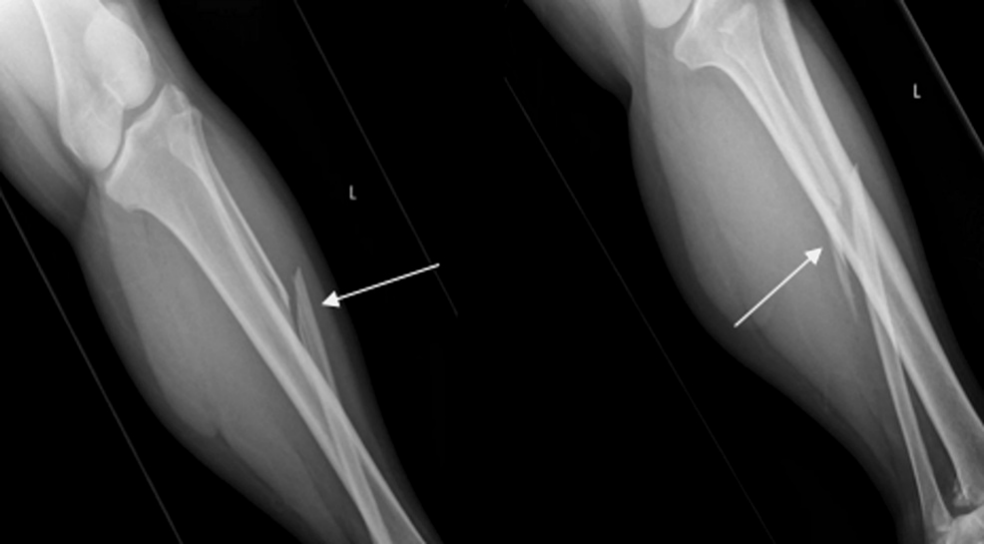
Left tibia/fibula anterioposterior/lateral X-ray images depicting a fracture of the proximal third of fibula
